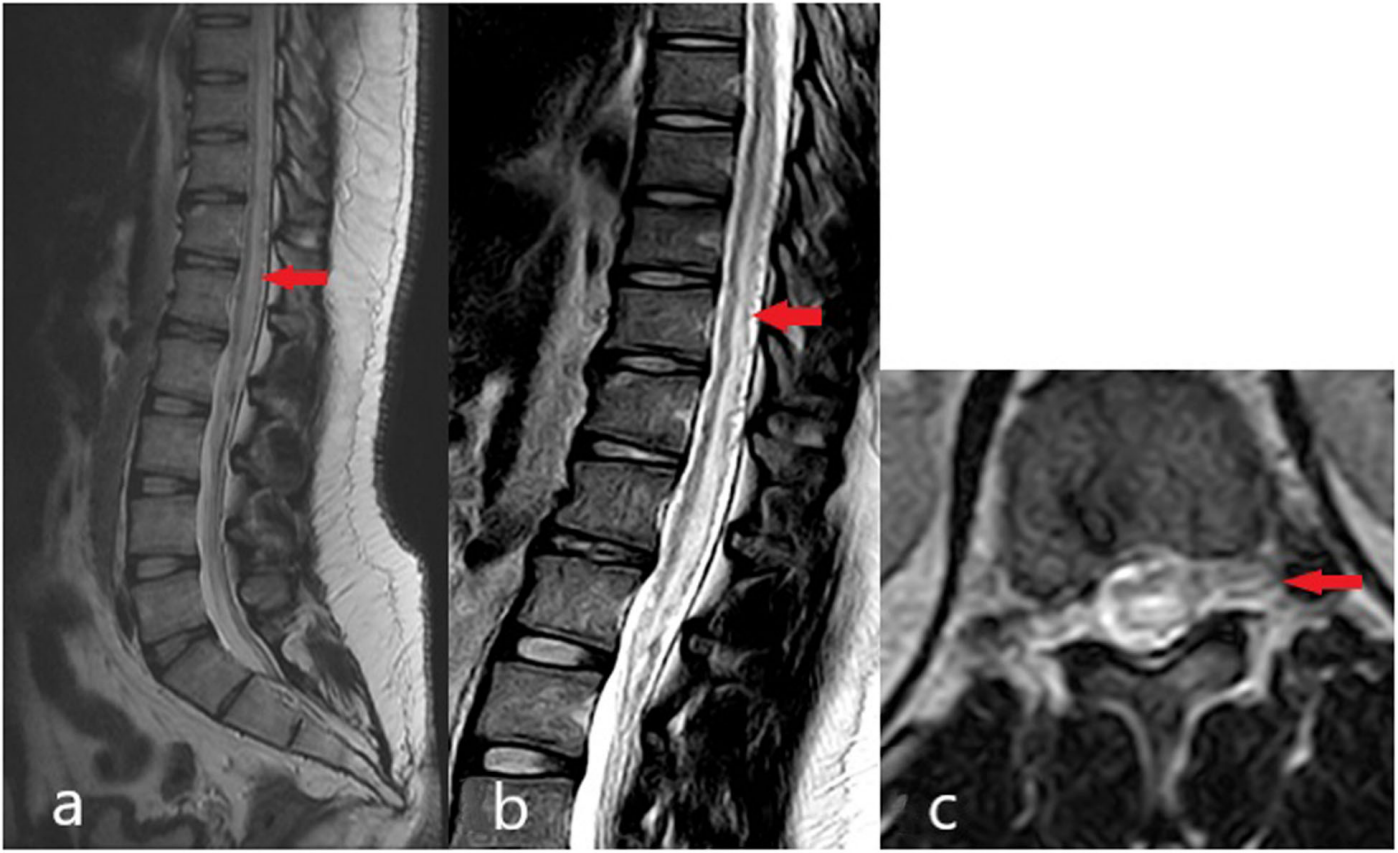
a and b MRI of patient 3 demonstrated T2 hyperintense intramedullary lesion with patchy enhancement extending from T7 to L1 (red arrows) and features suspicious of SDAVF with the dilated vessels seen at the thoracolumbar spinal cord (red arrows). c. MRI which clearly shows the dilated vessels and the feeding vessel (red arrow)
